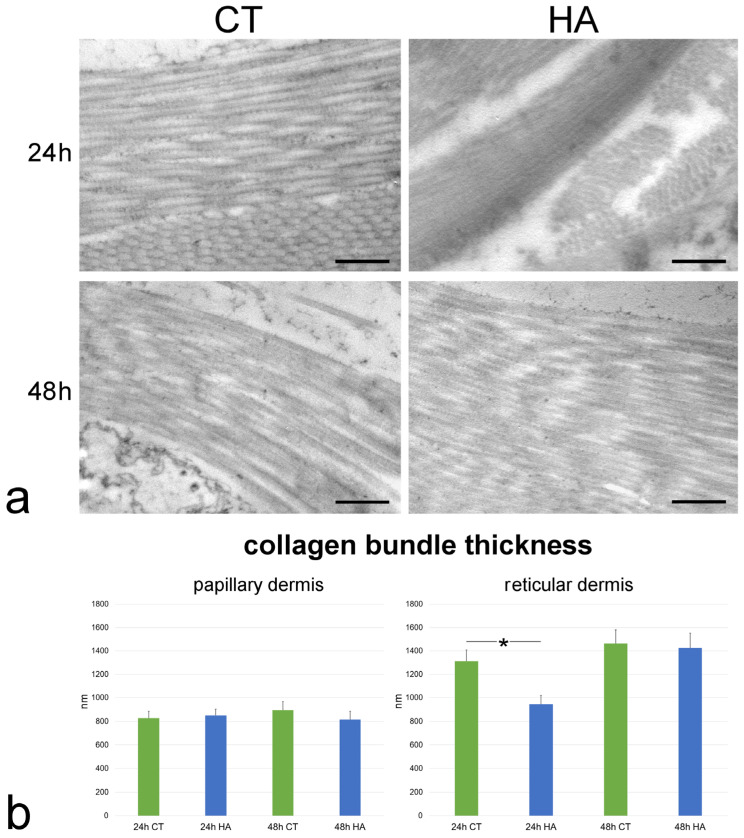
(a) TEM images showing collagen bundles in the reticular dermis of control (CT) and hyaluronic acid (HA)-treated skin. Bars, 500 nm. (b) Mean ± SE values (*n* = 40) of collagen bundle thickness in papillary and reticular dermis of control (CT) and hyaluronic acid-treated (HA) skin samples at 24 and 48 h. The asterisk (*) indicates a statistically significant difference between HA-treated samples and the relative CT.
